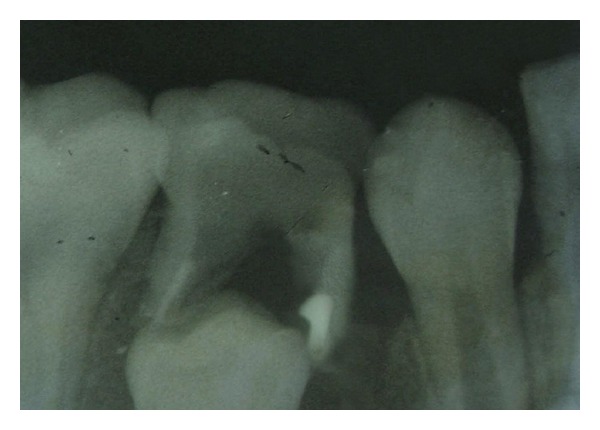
Intraoral periapical radiograph showing the radiopaque foreign body.
